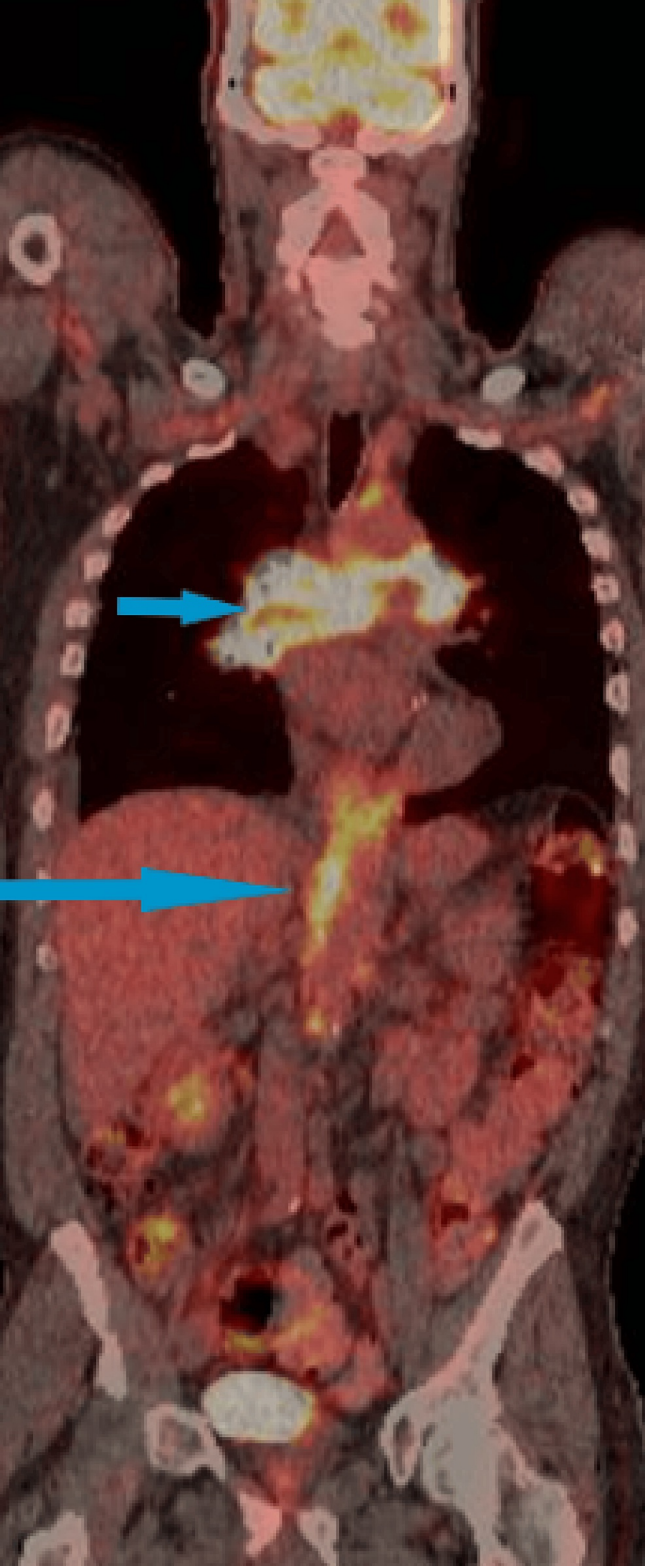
Fludeoxyglucose-18 (FDG) positron emission tomography (PET) scan. Blue arrows point to increased FDG uptake in both lung hila more on the right side and in soft tissue extending around the descending aorta at the level of the diaphragm. There was increased FDG uptake in the lung hila (SUV max = 13.5) and in soft tissue extending around the descending aorta with an SUV max of 12.0 at the level of left hilum and 12.5 at the level of the diaphragm. SUV max: maximum standardized uptake value.
